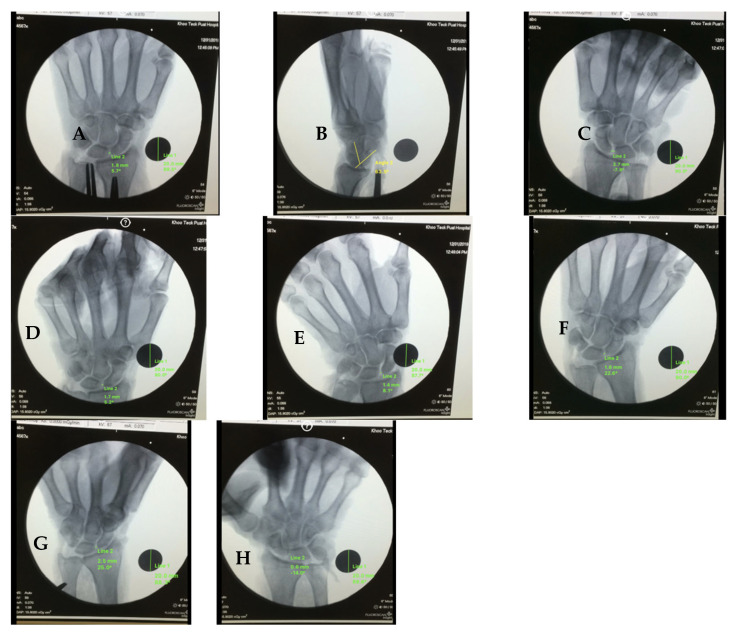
Representative radiographs showing SL widening. (A): PA neutral; (B): Lateral (SL angle); (C): Clenched fist (AP); (D): Clenched fist (PA); (E): PA ulnar deviation; (F): PA radial deviation; (G): PA wrist flexion; (H): PA wrist extension.
